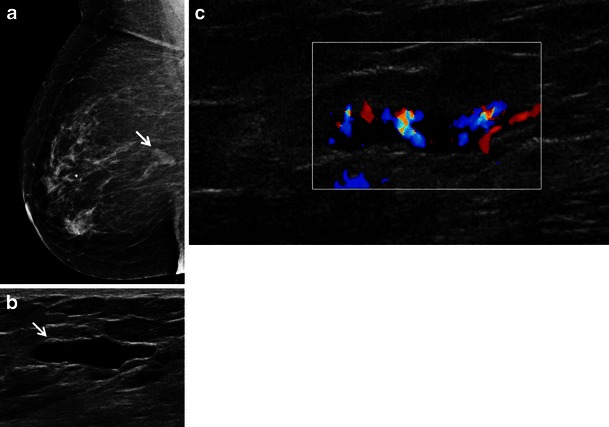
A 67-year-old woman with a history of marginal zone B-cell lymphoma (mucosa associated lymphoid tissue [MALT] lymphoma) presents with screening mammogram detected new mass in the right breast. a Right medio-lateral oblique mammogram demonstrates a lobulated circumscribed isodense mass in the upper right breast posterior depth (*arrow*). b, c Ultrasound shows an anechoic pseudocystic mass without posterior enhancement (*arrow*) which shows marked increased vascularity on colour Doppler evaluation indicating this is a solid mass
